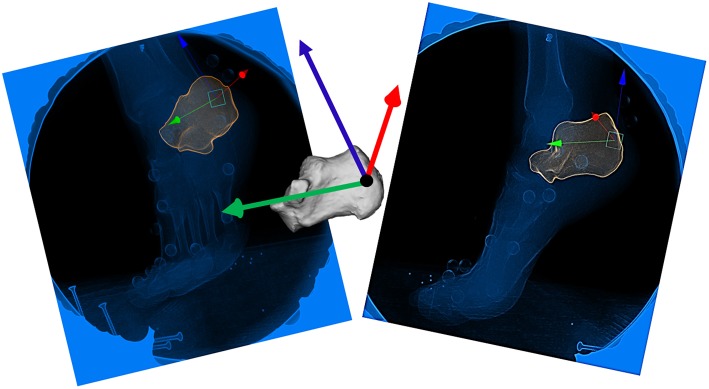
Scientific Rotoscoping: Depiction of the manual alignment of the calcaneus just prior to toe off. Each bone is aligned with the bony landmarks visible on the x-ray images in both cameras (camera 1, left and camera 2, right). Once the 3D bone is simultaneously aligned within both camera 1 and camera 2, it is considered “tracked” for that frame.
